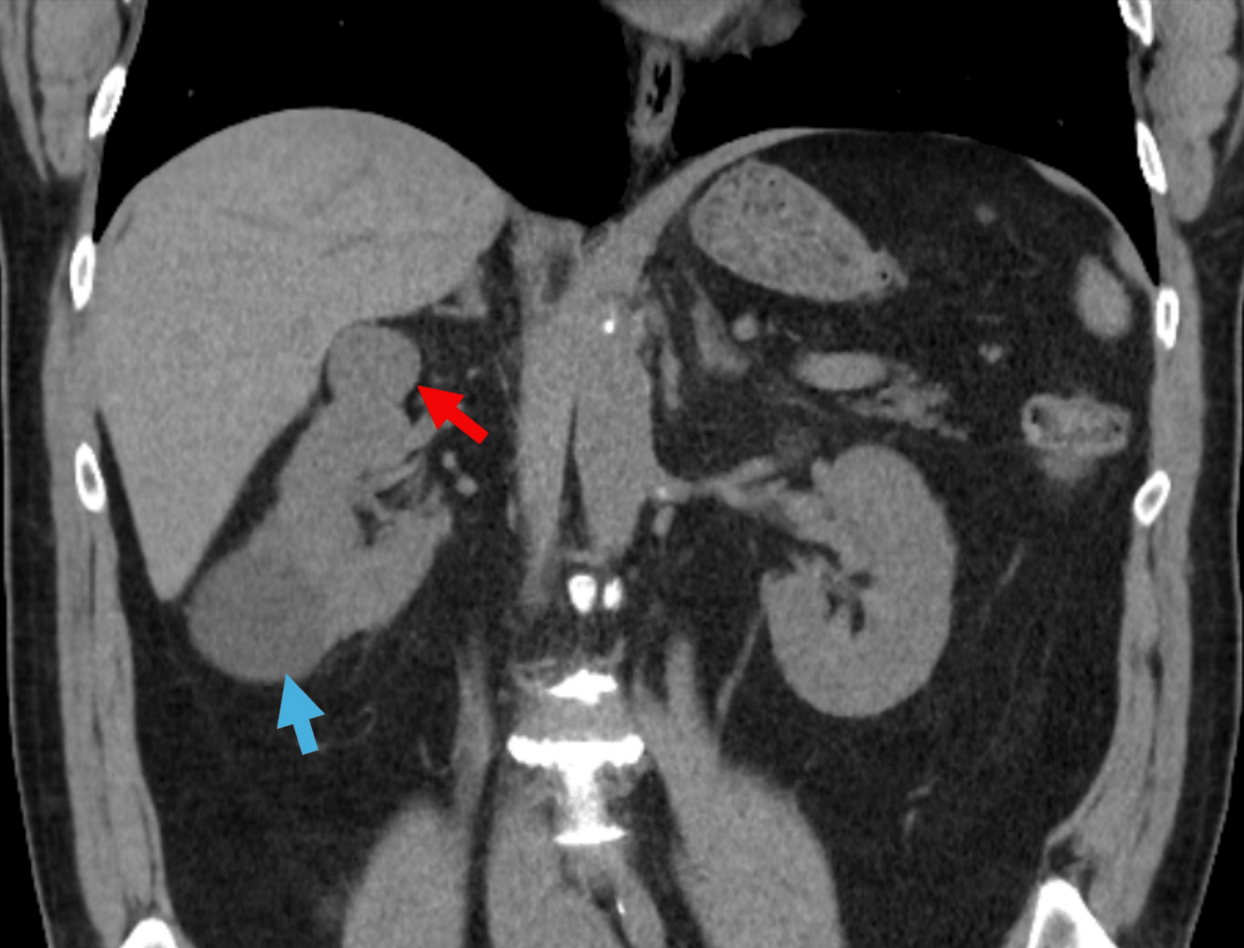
CT abdomen (coronal view) demonstrating a 3.1 cm, enhancing, right upper pole renal mass (red arrow) and a 5.2 cm, large, lower pole simple cyst (blue arrow)
